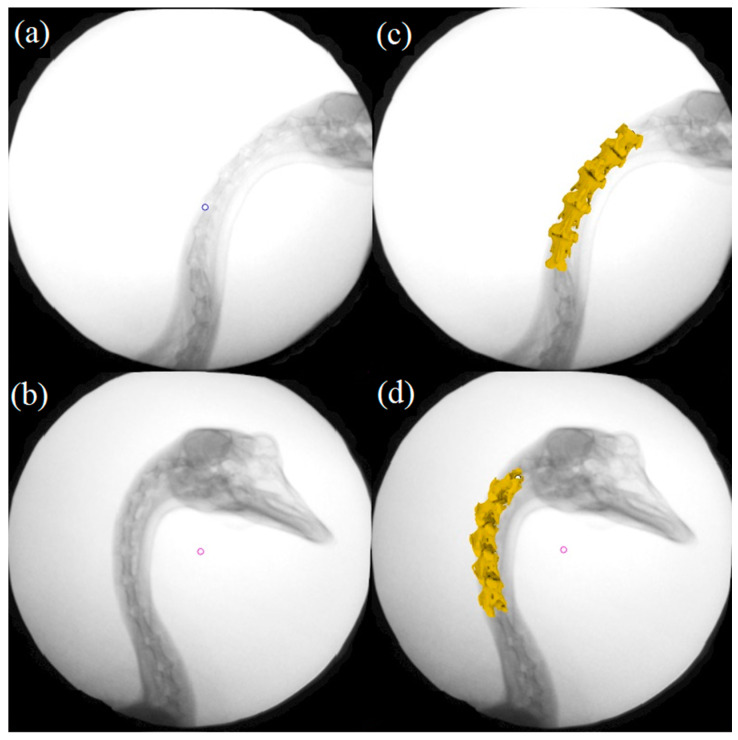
Relationship between model and radiograph positions before and after alignment: (a,b) X-ray images before alignment, (c,d) X-ray images after alignment.
